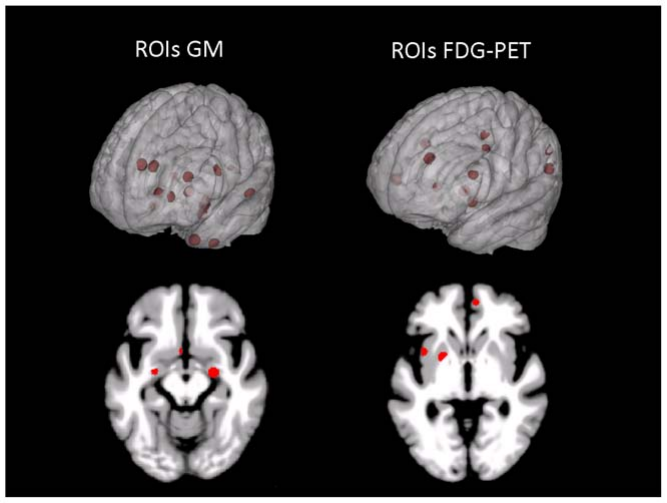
Regions of interest extracted from gray matter (left) and FDG-PET (right) data for AD and FTLD patients and used for support vector machine classification projected onto a glass brain (top) and onto an axial slice (bottom). AD Alzheimer's disease, FDG-PET [F18]fluorodeoxyglucose positron emission tomography, FTLD frontotemporal lobar degeneration, GM gray matter, ROIs regions of interest.
